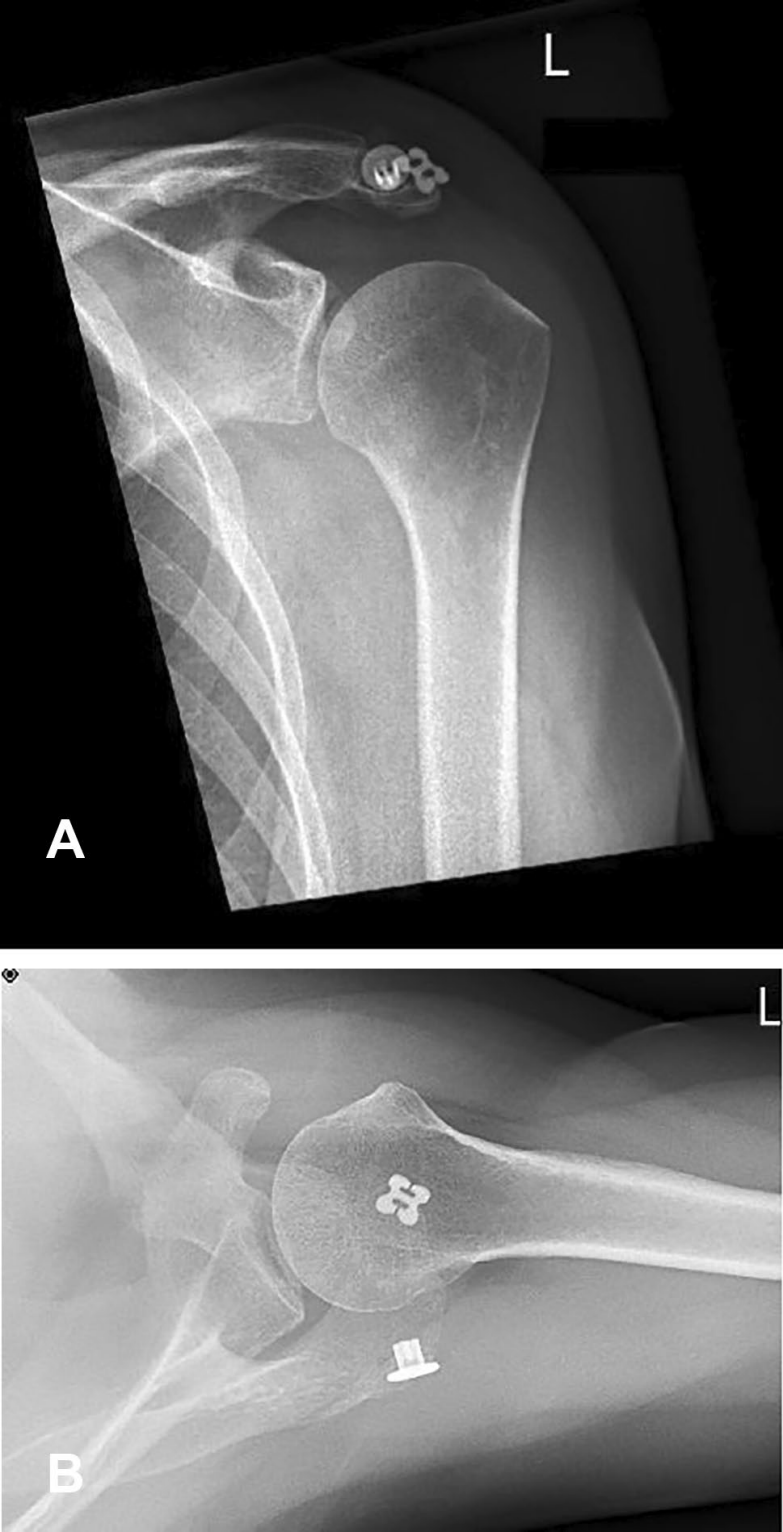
A–B Anteroposterior and axillary view radiographs at the 12-month follow-up
